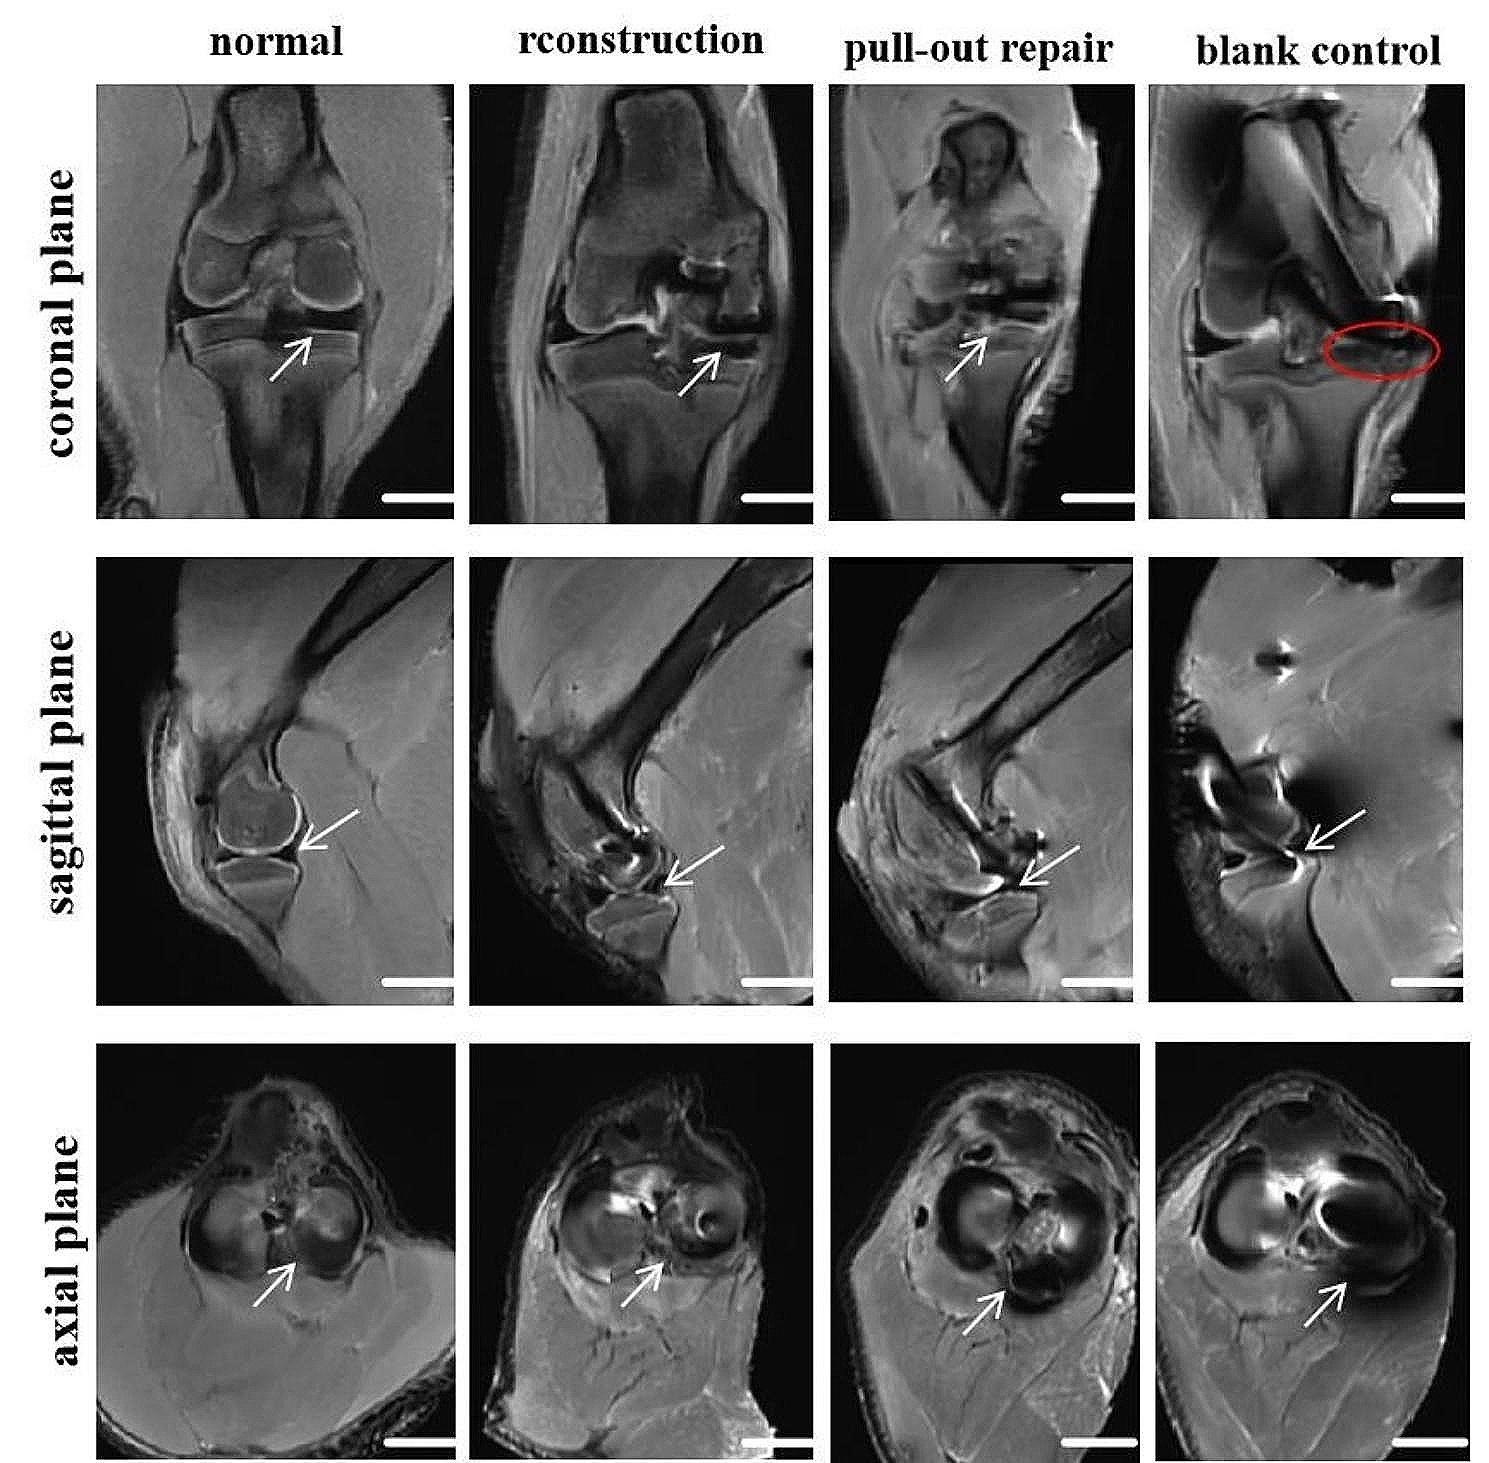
Coronal, sagittal, and axial MRI. White arrows indicate the MMPP. Red circles indicate complete extrusion and disappearance of the MM. Scale bar = 1 cm
